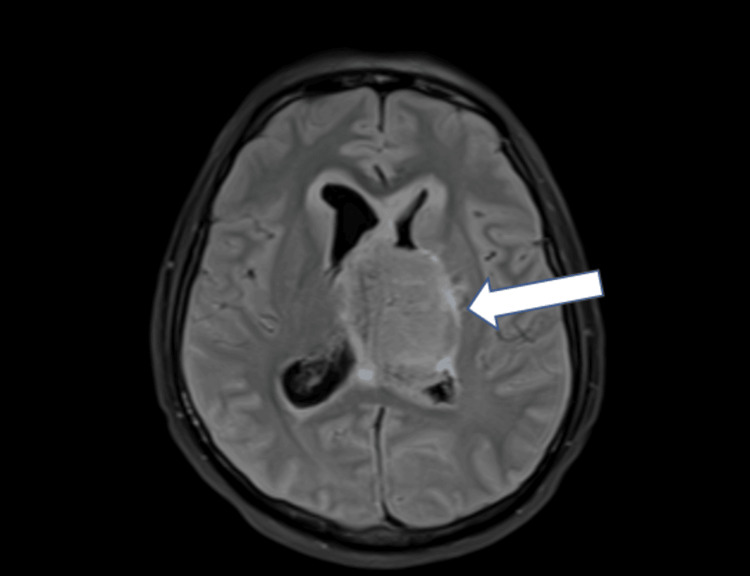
An axial image of brain MRI showing a large, well-defined lesion (white arrow) predominantly involving the third ventricle with compression of the lateral ventricles bilaterally and evidence of obstructive hydrocephalus. Adjacent brain parenchyma shows mass effect, including mild midline displacement MRI: magnetic resonance imaging
